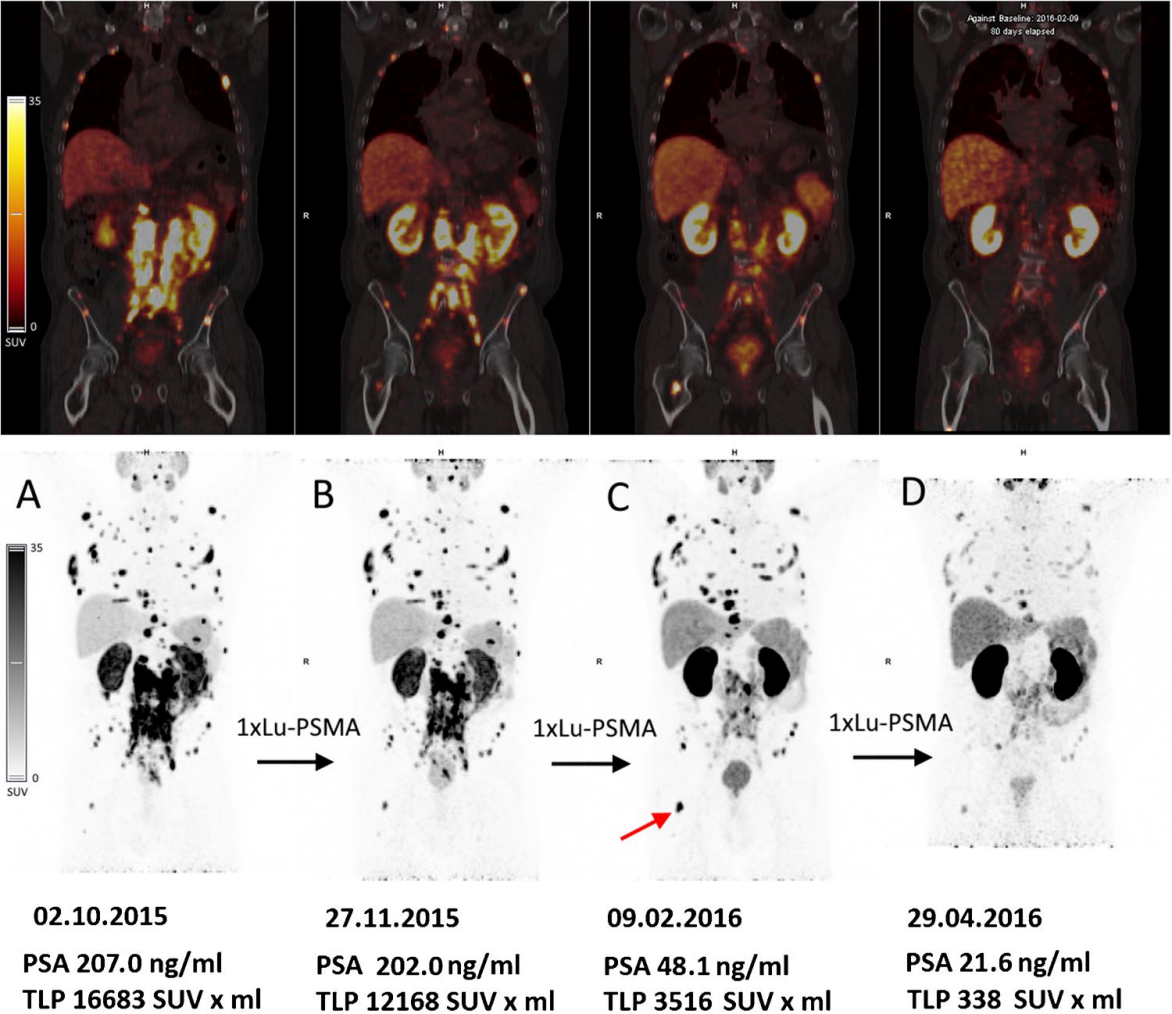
Example of a 61-year-old patient with advanced mCRPC, presenting multiple bone and abdominal lymph node metastases, treated with [^177^Lu]Lu-PSMA I&T. Sub panel A represents baseline [^68^Ga]Ga-PSMA PET/CT scan. Sub panel B shows [68Ga]Ga-PSMA PET/CT scan after 1 cycle of PSMA RLT. After 3 cycles of PSMA RLT, the patient achieved the best biochemical and radiographic partial response D. After the second cycle a transient increase of [^68^Ga]Ga-PSMA uptake was observed in some metastatic lesions, e.g., in the right femur (C, red arrow) indicating a “flare effect,” which resolved after the subsequent cycle. The OS from the beginning of RLT was 18 months. mCRPC, metastatic castration resistant prostate cancer
